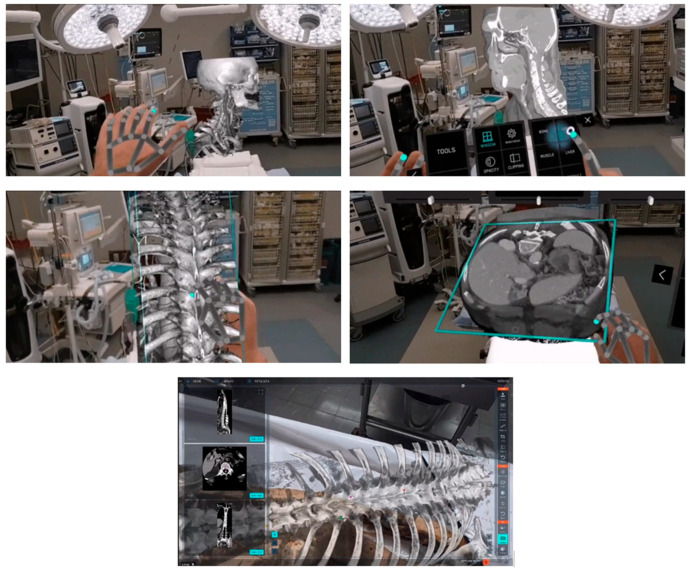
Virtual and augmented reality in healthcare is revolutionizing surgical procedures by providing advanced intraoperative scans that create detailed and high-resolution 3D models. These sophisticated models come equipped with automatic spine support, facilitating precise planning and navigation throughout the surgical process. High-resolution cameras play a crucial role by accurately detecting patient markers, ensuring the surgical team has clear and real-time identification of key anatomical features. Additionally, integrating 3D cone-beam Computed Tomography (CT) with live video images enhances the surgeon’s view, allowing for more effective decision-making and improved patient outcomes during complex operations.
